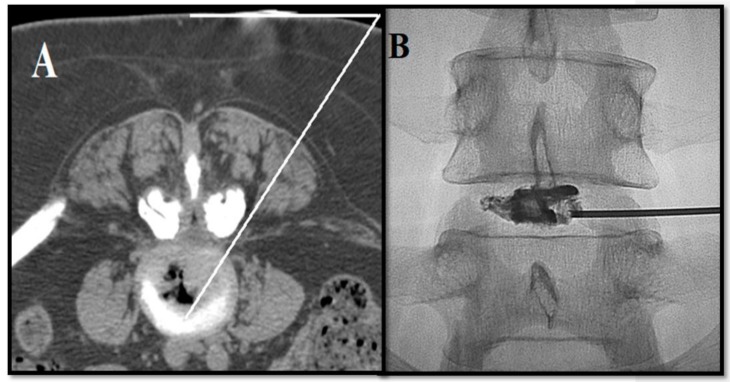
(A) Needle trajectory obtained via CT guidance. (B) Patient with symptomatic L4–L5 intervertebral disc herniation. Antero-posterior fluoroscopic view: discography shows correct needle positioning.
